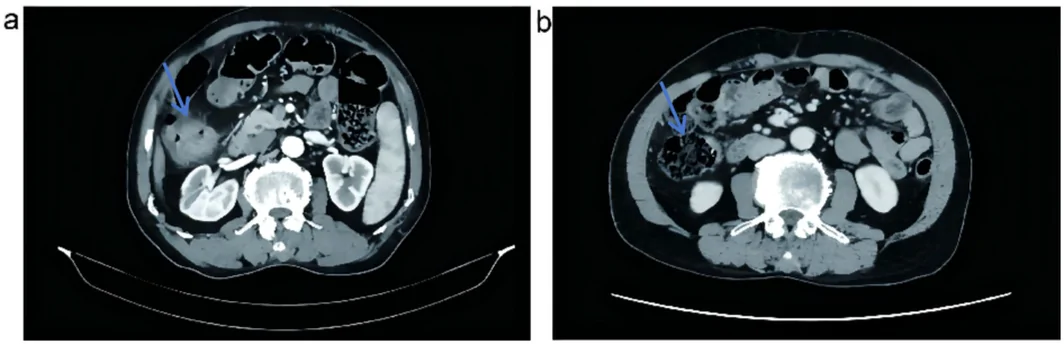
CT scan of the proband's father showed considerable tumor regression. (a) Before treatment, (b) After treatment. Blue arrows indicate the primary lesion(s) in each panel to show tumor size and extent before and after immunotherapy.
